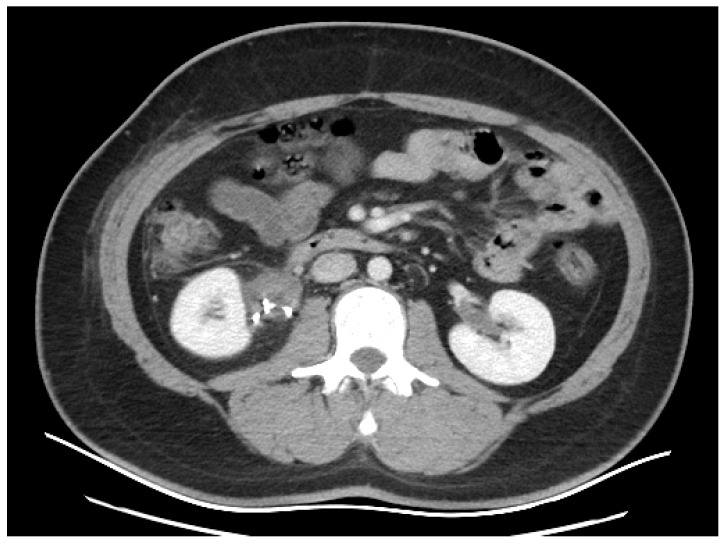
Thirty-days follow-up CT scan of the abdomen.
